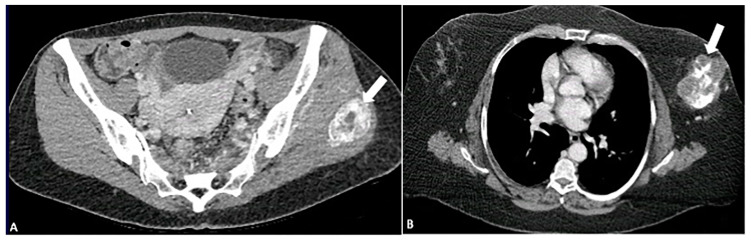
Pattern of calcification in myositis ossificans (A) versus osteosarcoma (B) on CT images. In myositis ossificans, calcifications (arrow) are organized and densest at the periphery, a phenomenon known as the “zoning phenomenon”. In contrast, osteosarcoma shows disorganized calcifications that are densest in the center (arrow). The image was obtained from the University of Washington Medical Imaging database with the required permissions.
